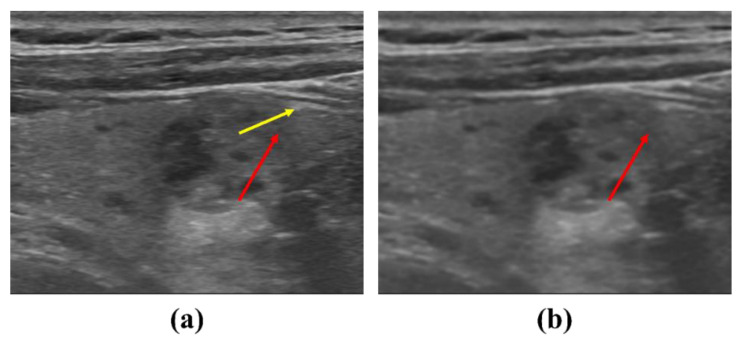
Ultrasound images to observe changes in the margin of the thyroid nodule: (a) noisy image and (b) NLM denoising with high smoothing. The red arrow means the actual exact nodule margin, and the yellow arrow means the margin that can be misunderstood.
